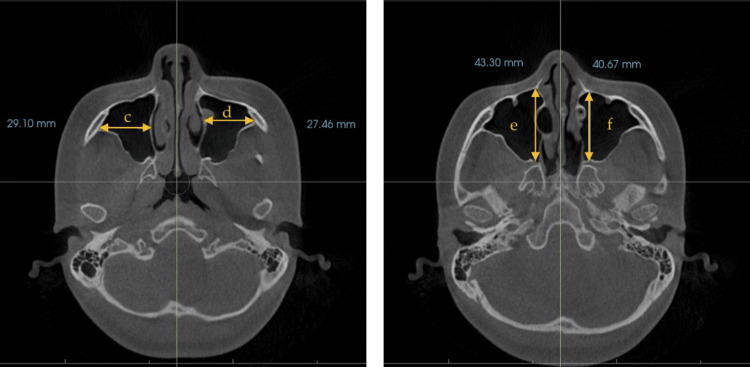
Measurement of maxillary sinus length and width using an axial cone-beam computed tomography (CBCT) image. The figure on the left is A, and the figure on the right is B. A: measurement of maxillary sinus width using an axial CBCT image. This parameter comprised the longest distance from the most medial wall of the sinus to the most lateral wall. c: right maxillary sinus width; d: left maxillary sinus width B: measurement of maxillary sinus length using an axial CBCT image. This parameter comprised the longest distance from the most anterior point to the most posterior point of the medial wall. e: right maxillary sinus length; f: left maxillary sinus length
